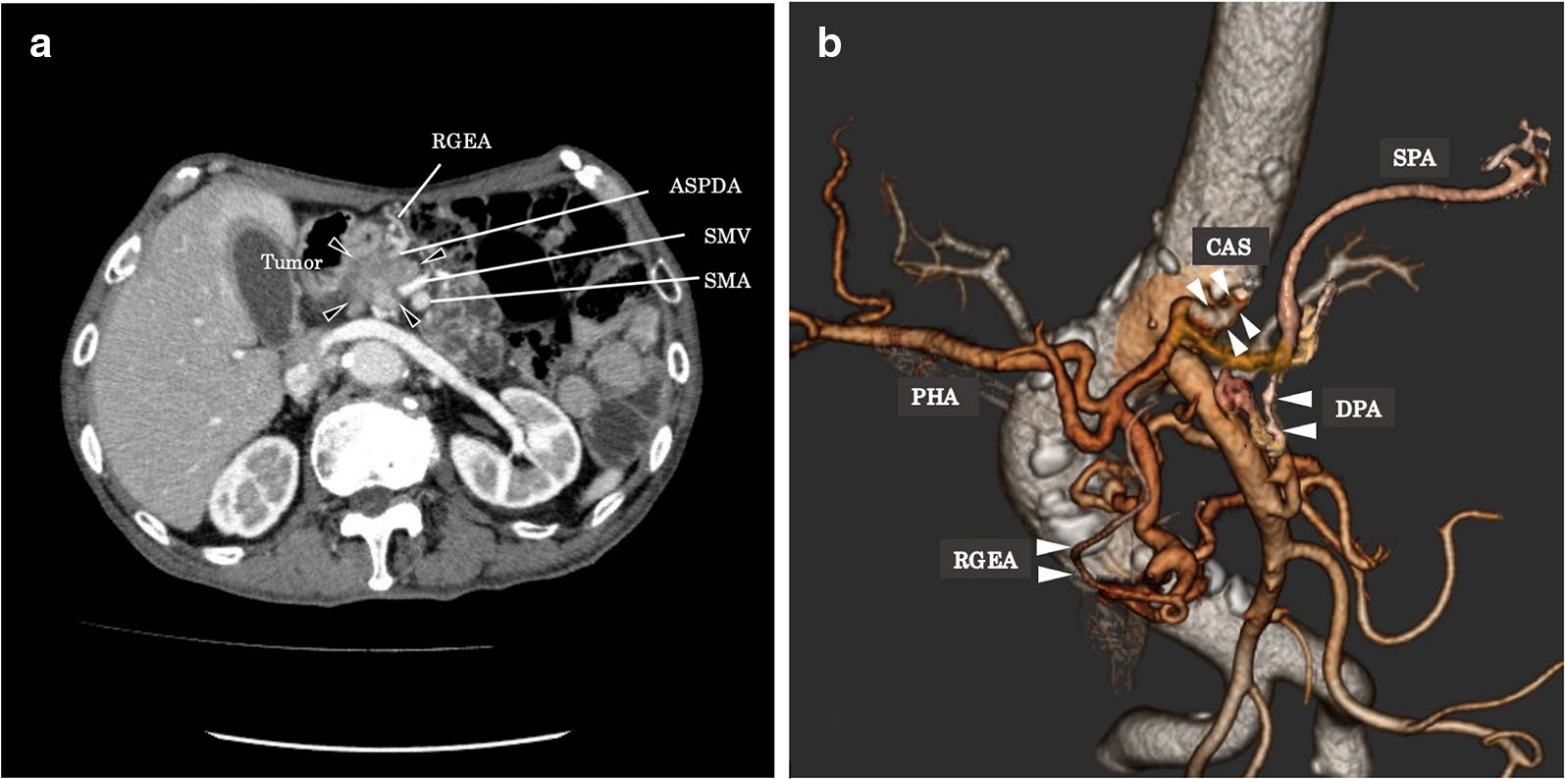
Axial view and three-dimensional reconstruction using a preoperative abdominal contrast-enhanced computed tomography. a Iso-dense mass (arrowheads) in the pancreatic head with attachment on the anterior superior pancreaticoduodenal artery (ASPDA). b Celiac artery stenosis (CAS) and branch from the superior mesenteric artery (SMA) to the splenic artery (SPA) via the dorsal pancreatic artery (DPA) before pancreaticoduodenectomy
